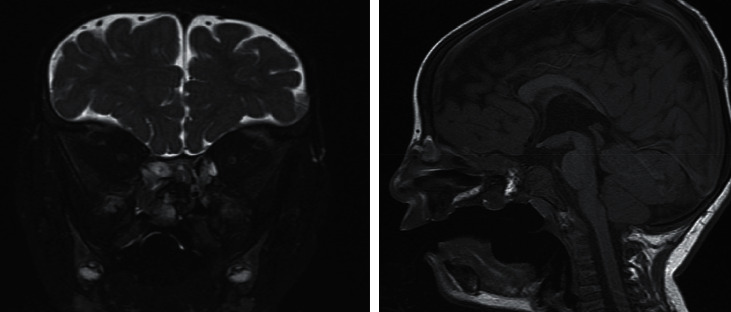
MRI of the head and neck showing right nasal mass and nasion mass which is hypointense in T1 and isointense in T2.
